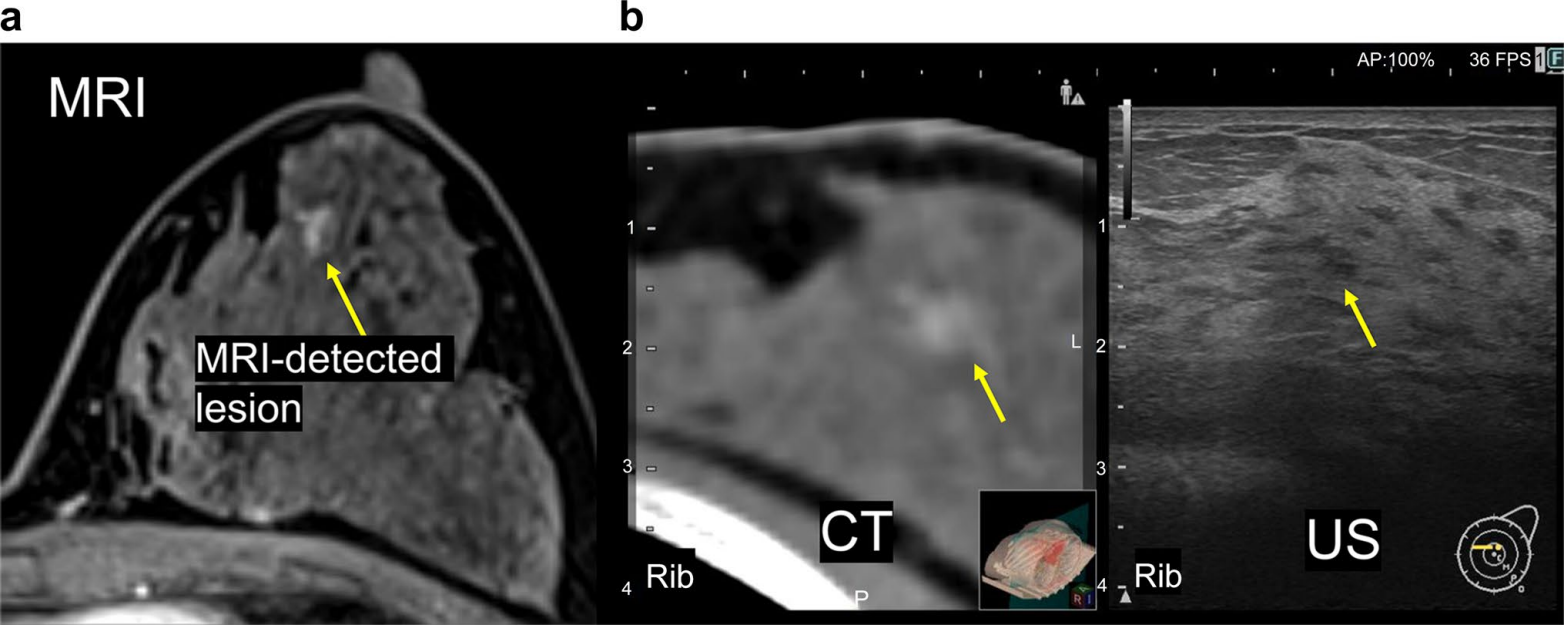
MRI-detected lesion identified with RVS^®^ in a patient with HBOC syndrome. a MRI-detected lesion in the unaffected breast of a patient with HBOC syndrome (right breast cancer). b After confirming the position of the MRI-detected lesion on the CT image, we fused the CT image with the ultrasound image using RVS^®^. The images created by RVS^®^ clearly identified the MRI-detected lesion (yellow arrow) with improved CT-ultrasonography fusion imaging, which was difficult to perform with ultrasonography alone
